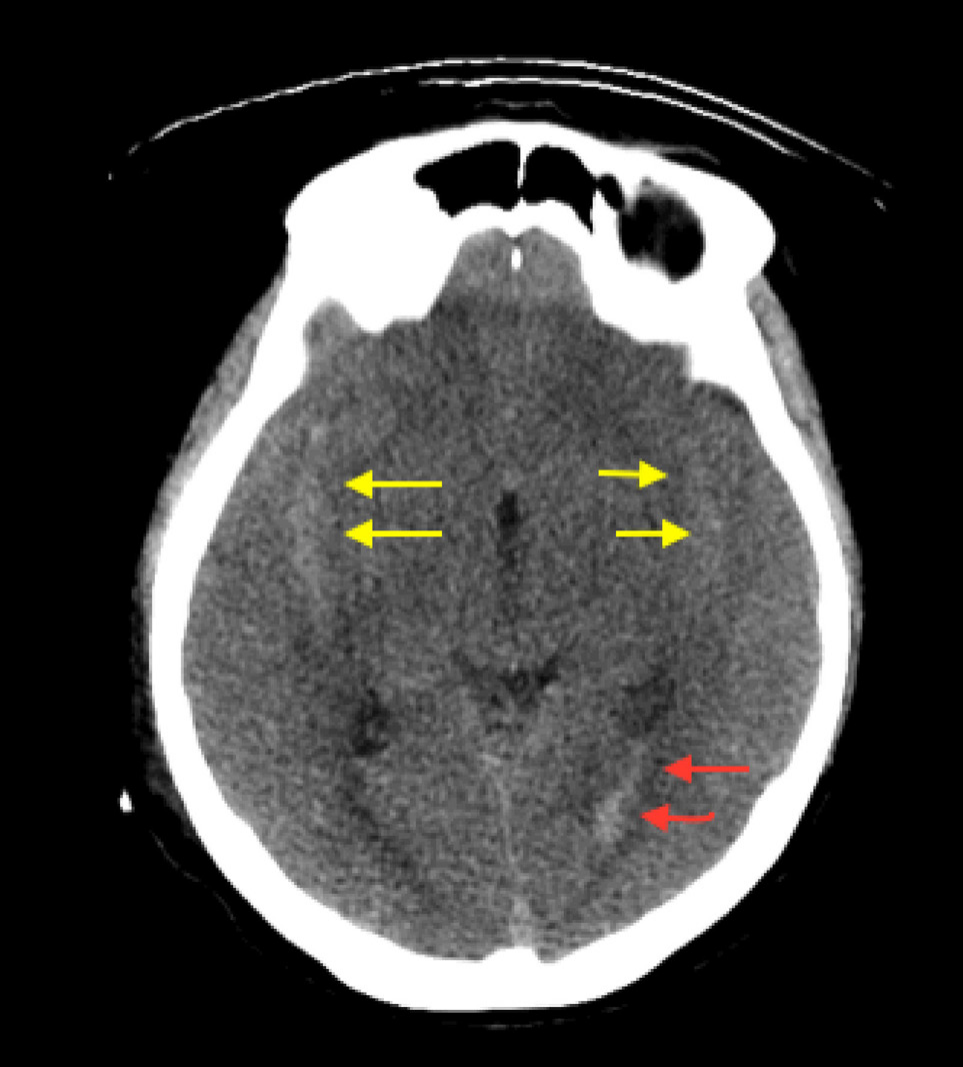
Axial section CT demonstrating diffuse brain edema evident by effacement of cortical sulci and the subarachnoid hemorrhage in both Sylvian fissures (yellow arrows) with seeding into the ventricular system (red arrows).
